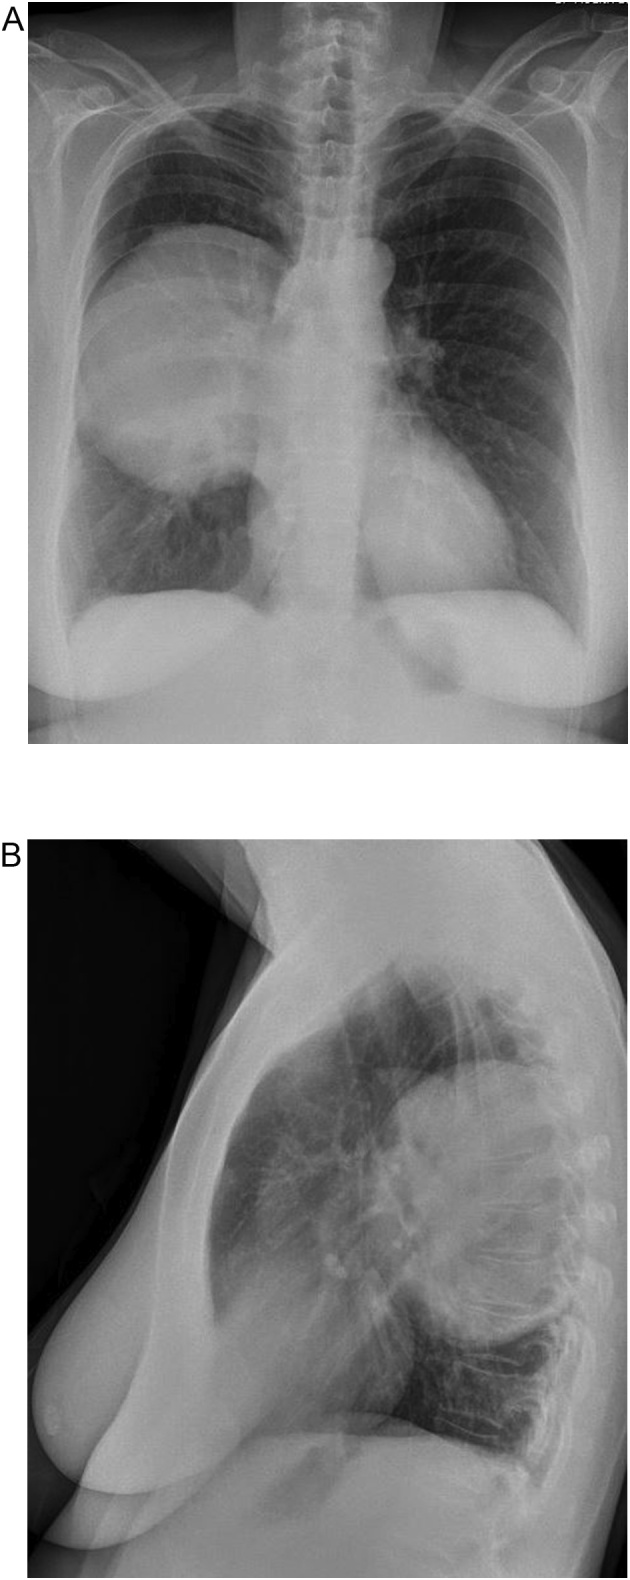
Chest radiograph demonstrating a large round opacity in the right chest (1A: Anterior-posterior view, 1B: Lateral view).
